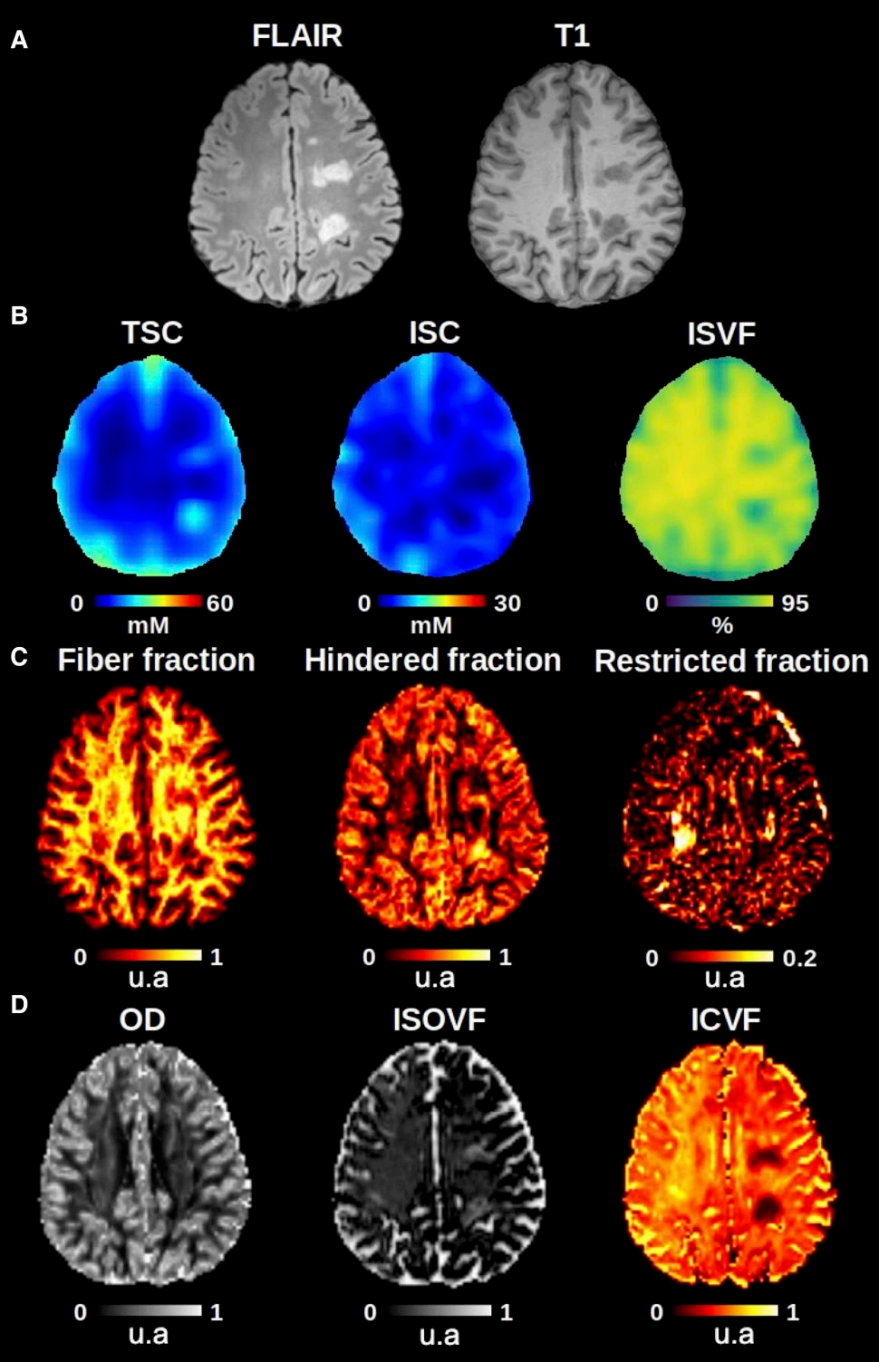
Examples of the MRI sequences and metrics analysed in this study. (A) Shows the T1-weighted and FLAIR images used to identify MS lesion. (B) The ^23^Na extracted maps are represented, including TSC, ISC and ISVF. (C and D) Show the DBSI maps (fibre fraction, hindered fraction and restricted fraction) and NODDI maps (OD, ISOVF and ICVF), respectively. In the selected images, 2 examples of MS lesions ≥0.41 cm^3^ are shown. FLAIR = fluid attenuated inversion recovery; TSC = total sodium concentration; ISC = intracellular sodium concentration; ISVF = intracellular sodium volume fraction; OD = orientation dispersion; ISOVF = isotropic volume fraction; ICVF = intracellular volume fraction.
